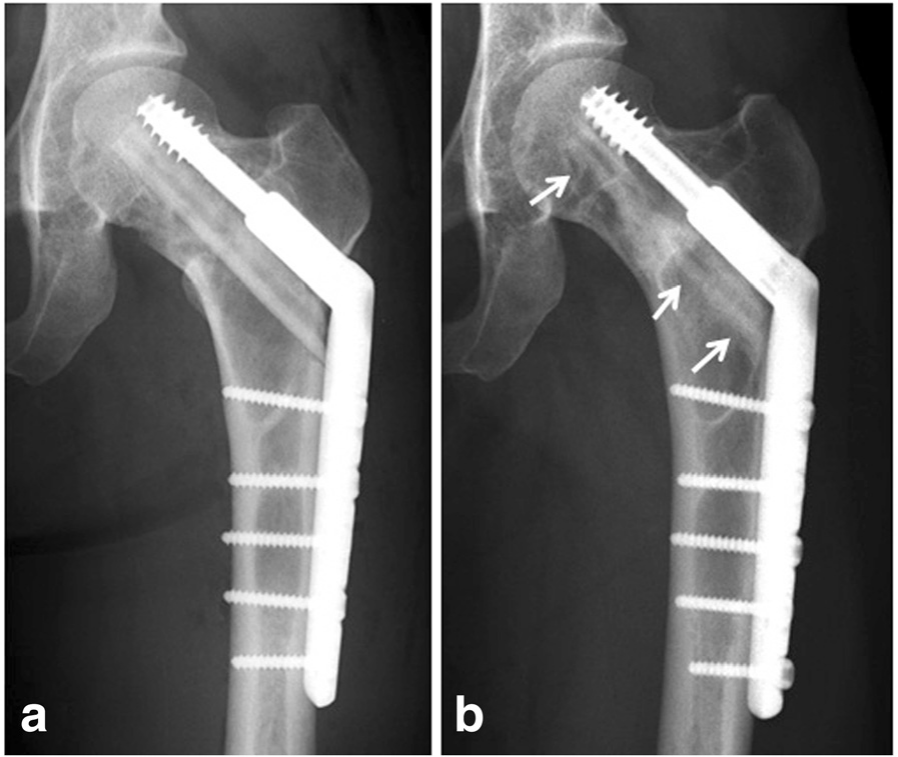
Patient 4. X-ray at the time of surgery after fixation with a compression hip screw (a). Nine years after surgery, a grafted fibular strut was well preserved (b, *arrows*)
